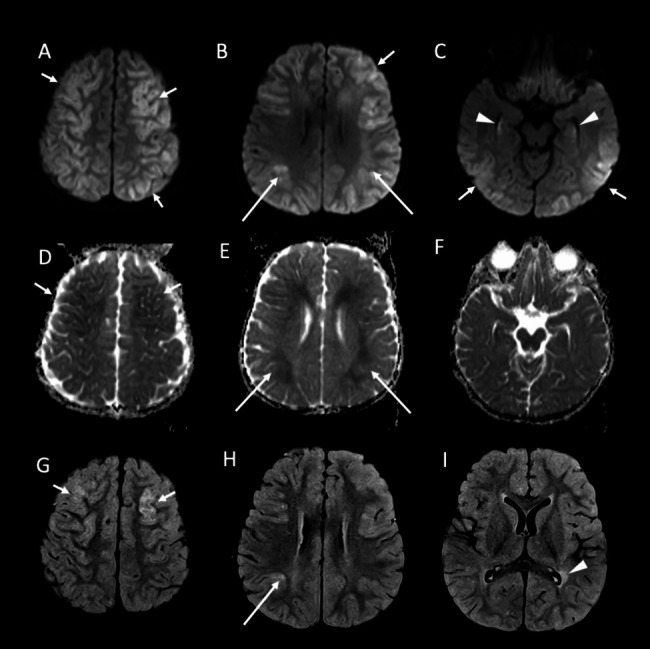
Brain MRI characteristic of PEPCK-C deficiency in the proband (Patient 1.2) of Family 1. (A-C) Axial diffusion-weighted images; (D-F) ADC map images; and (G-I) axial FLAIR images: There are bilateral areas of increased cortical signal intensity in diffusion-weighted images in the cerebral hemispheres (short arrows, A-C). Some of these areas in the superior frontal and parietal lobes show diffusion restriction (arrows in D) and FLAIR hyperintensity (for example, arrows in G). Diffusion restriction was also present in the genu of the corpus callosum (not shown) and in the hippocampi (arrowheads, C). There is diffusion restriction in the deep and subcortical white matter in the frontal and parietal lobes bilaterally with focal faint FLAIR hyperintensity in the right parietal lobe (long arrows in B, E, and H). Focal peri-trigonal white matter FLAIR hyperintensities were present without diffusion restriction (arrowhead, I)
